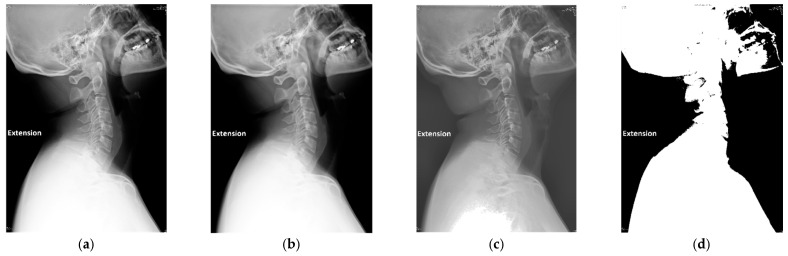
The result for the cervical spine radiography by different preprocessing. (a) Original image. (b) The median filtered image. (c) The image after contrast enhancement. (d) Binarized image.
